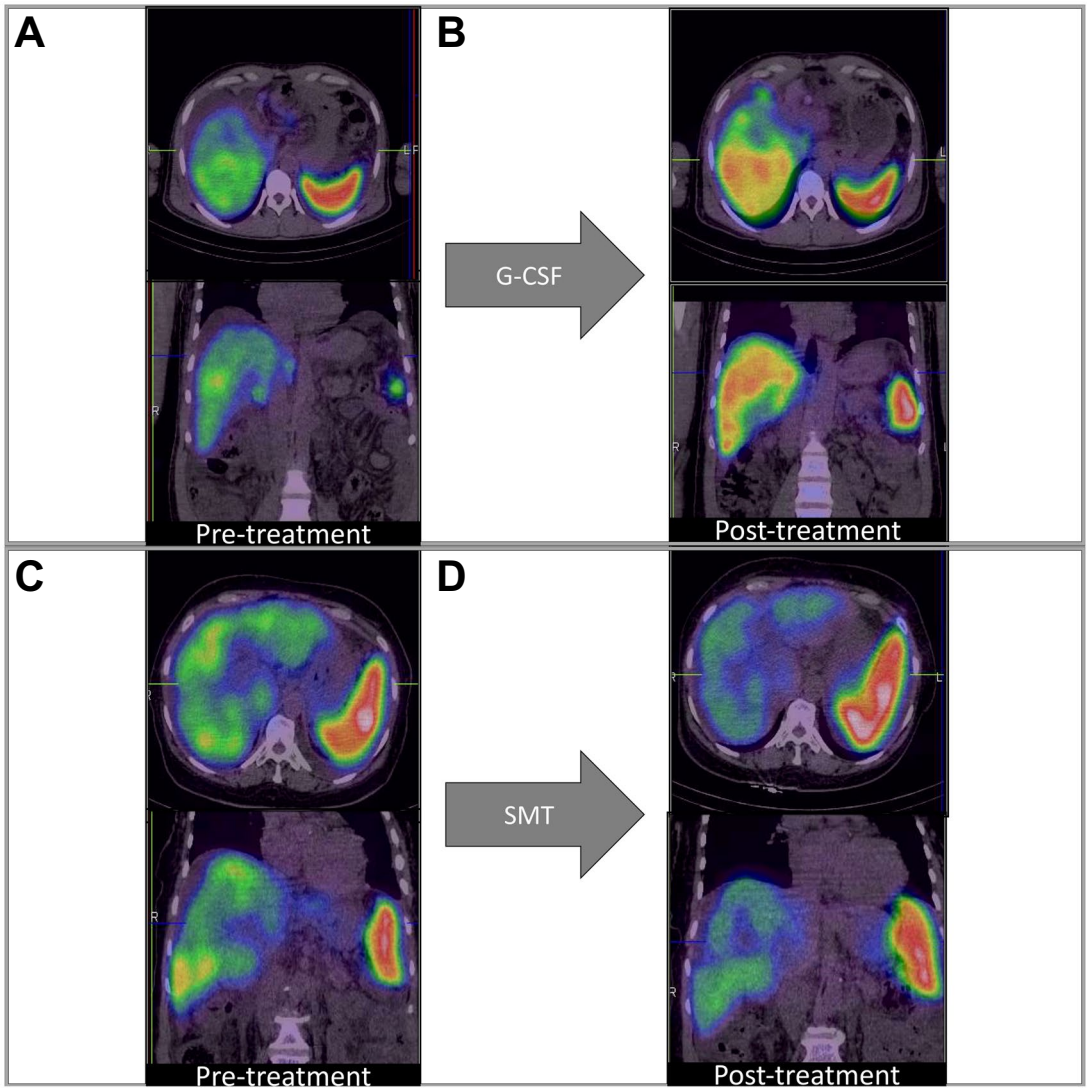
Q-SPECT/CT liver images with granulocyte colony-stimulating factor; G-CSF treatment [Panels (A,B)] and standard medical treatment (SMT) [Panels (C,D)] in representative DC patients. (A,B) Rise in %QLU (30 to 45%) and LV (964 to 1,016 cc) after G-CSF treatment (C,D) Fall in %QLU (41 to 36%) and LV (1,264 to 965 cc) after SMT treatment.
